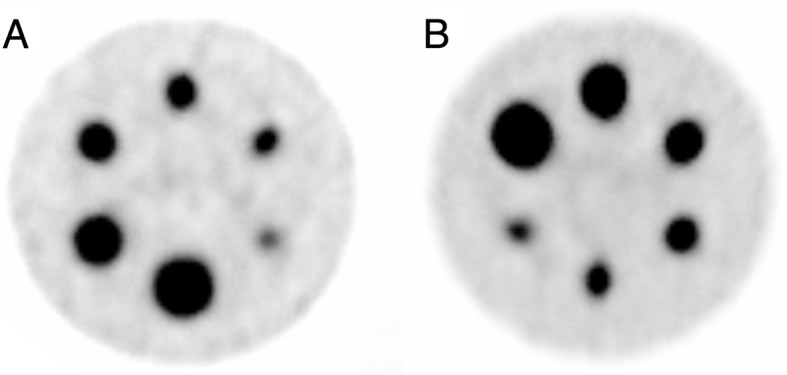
ASC-OSEM reconstructed SPECT images of ^161^Tb (a) and ^177^Lu (b) with hot-sphere-to-background ratios of 10 and 25, respectively. The color scale is normalized to each image separately
